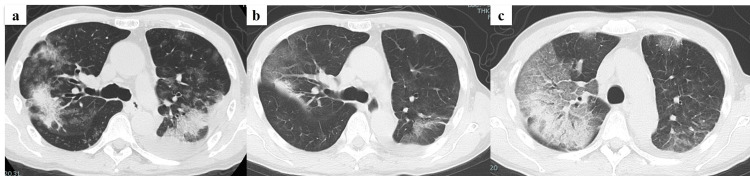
Computed tomography image of the chest. A 72-year-old male patient with lung adenocarcinoma presented with multiple lung metastases and pleurisy (Case 2). Osimertinib monotherapy was initially administered. On the 11th day of treatment, fever and respiratory failure were observed, and chest computed tomography (CT) was performed. A CT scan of the chest revealed that the metastatic lung tumor had shrunk. However, a newly developed, extensive frosted shadow was observed, mainly in the right upper lobe. Drug-induced lung injury was diagnosed, and osimertinib was discontinued. (a) Chest CT before the initiation of epidermal growth factor receptor-tyrosine kinase inhibitor therapy; (b) chest CT at interstitial lung disease onset
